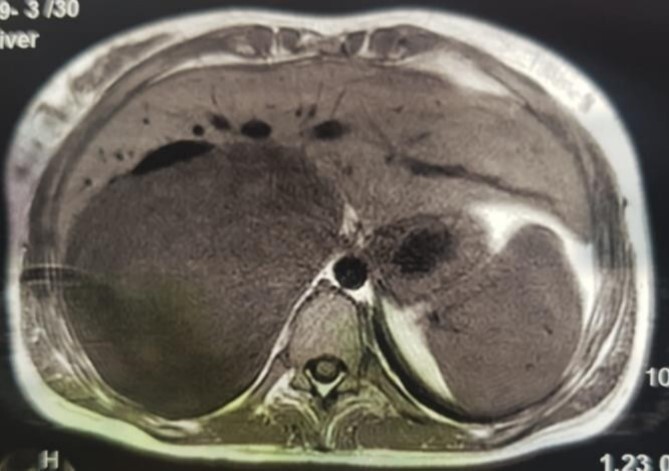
MRI, voluminous lesion of the right adrenal medulla in hyposignal T1.
